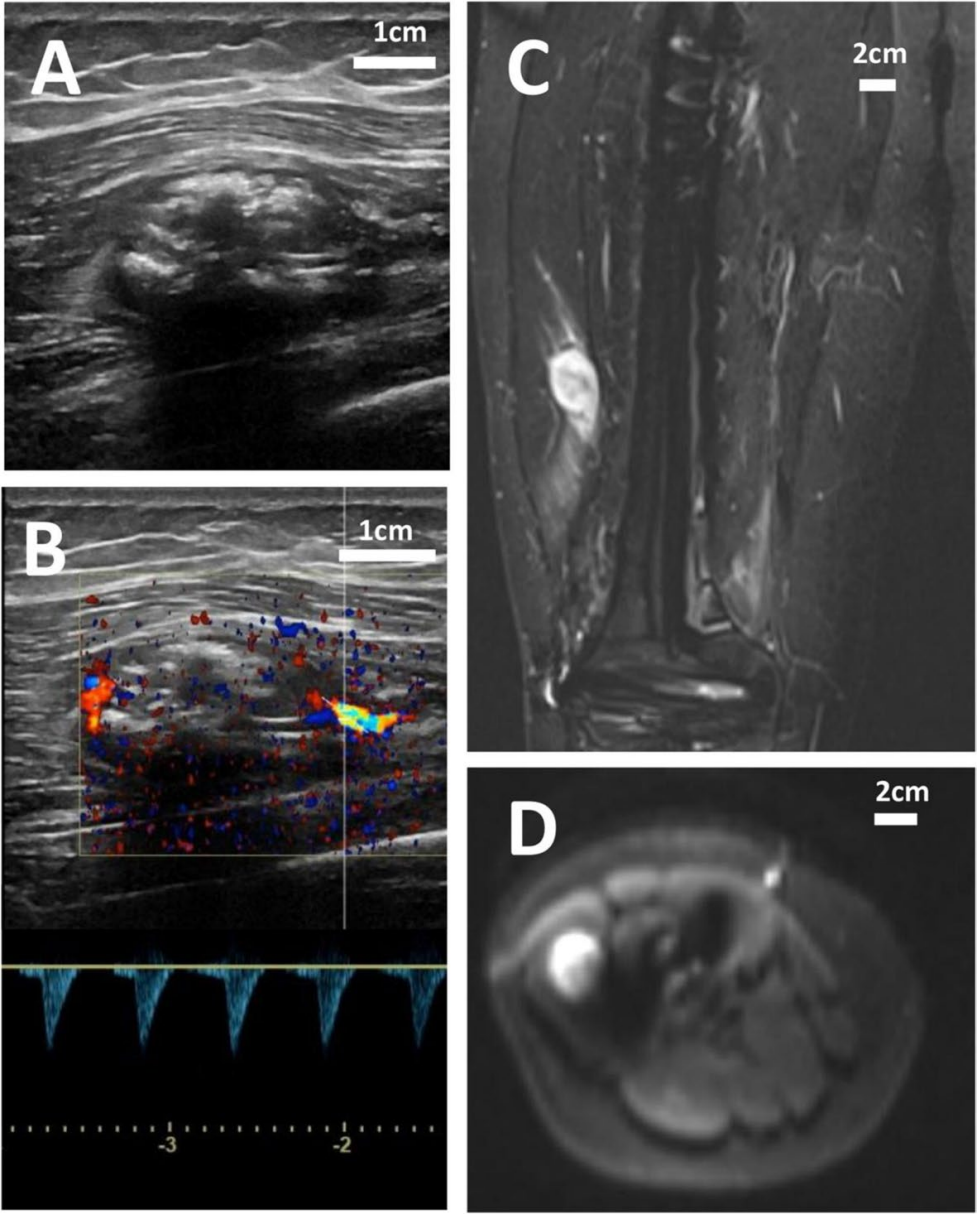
Representative imaging findings of the lesion on US and MRI. A Longitudinal US image demonstrating a heterogeneous echogenic mass. B Corresponding US image showing intramuscular localization with vascularity and calcifications. C MRI STIR sequence revealing perioperative high signal intensity. D Diffusion-weighted MRI exhibiting restricted diffusion within the nodule
